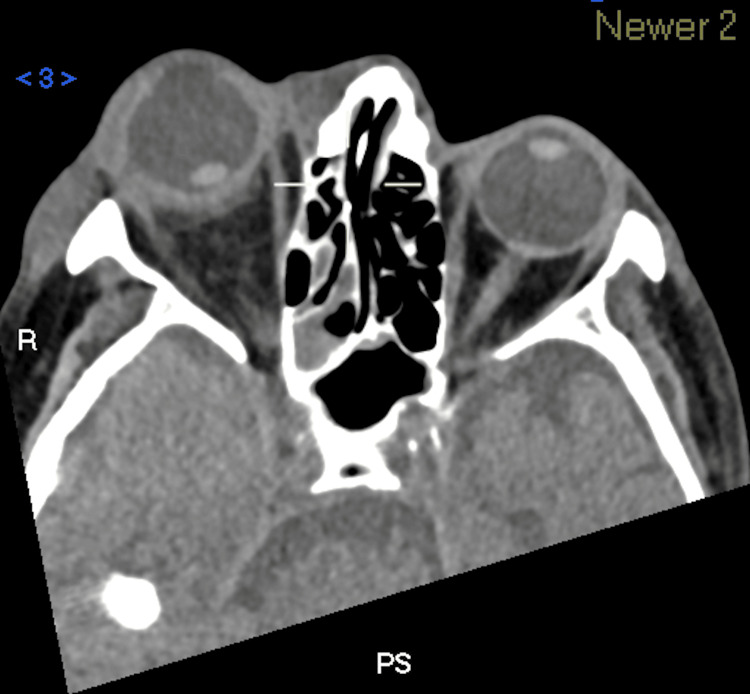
CT imaging demonstrating proptosis, pre-septal swelling, intraconal edema, and possible lens dislocation.
